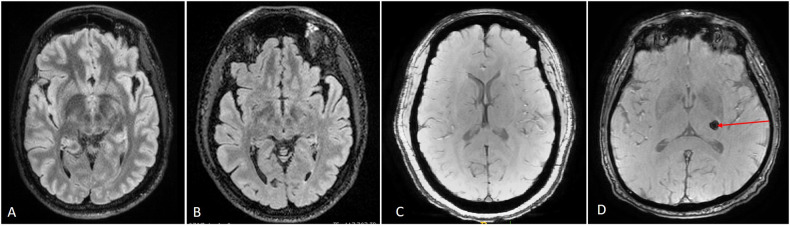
T2 FLAIR and SWI images. T2 FLAIR is a technique that accentuates the white matter hyper-intensities while nulling the signal from CSF (A, B). On T2 FLAIR sequences, the white matter is dark, the gray matter is bright, and the CSF in the ventricles is dark. This technique allows for subtle white matter hyperintensities to be detectable even in areas close to the ventricles. Susceptibility-weighted imaging (SWI) is a gradient echo technique that takes advantage of both phase and magnitude effects to accentuate the presence of ferromagnetic, paramagnetic, and diamagnetic compounds (C, D). Thus, SWI is used to identify microbleeds, blood products, and calcium. C is a standard SWI image and D. is from a patient with a small hemorrhage (red arrow) that is seen as a dark ring.
